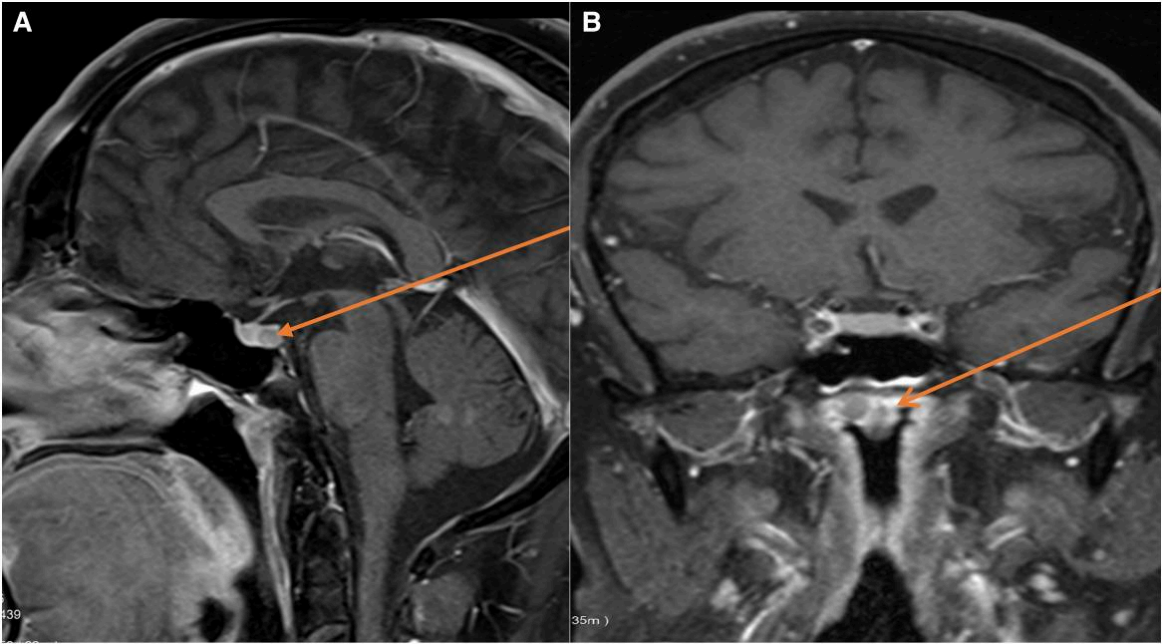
(A and B) Dynamic contrast-enhanced T1-weighted MRI of the pituitary gland demonstrating a small pituitary microadenoma. (A) Sagittal view. (B) Coronal view.
